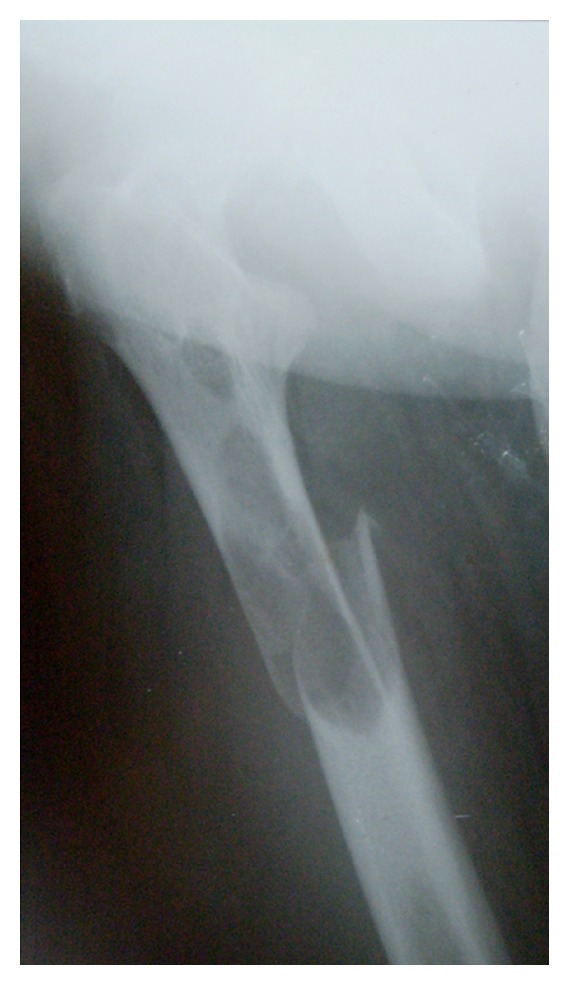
Radiographs of pathological subtrochanteric fracture of right femur.
